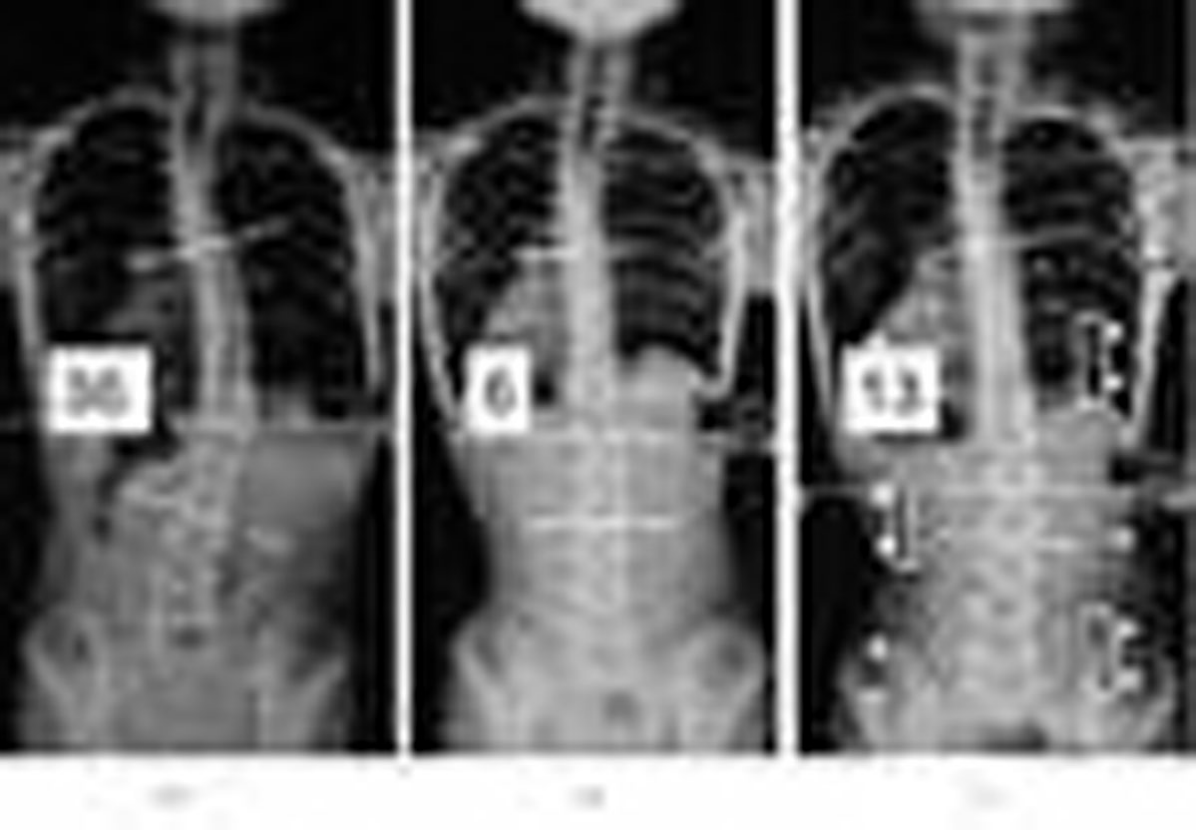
a: before treatment, b: first in-brace radiograph at the time of trial fitting, c: second in-brace radiograph after 2 months. Informed consent was obtained from the patient for the image(s) used in this study.
